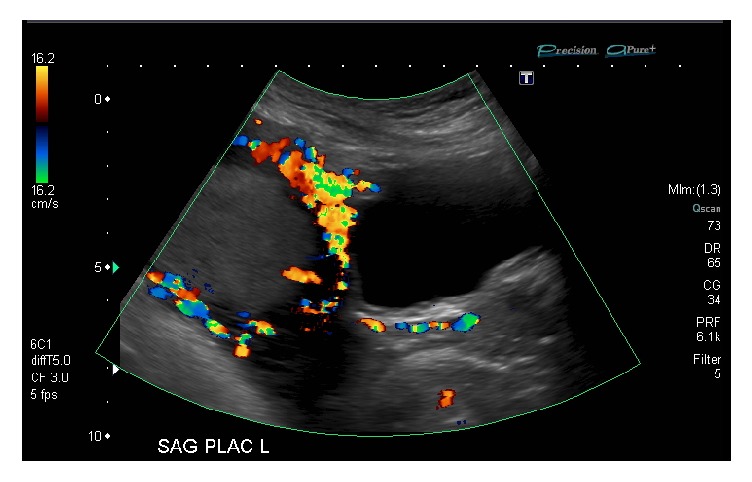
Doppler ultrasound in the sagittal plane at midline in the pelvis demonstrates turbulent peripheral vascularity in the placenta extending across the myometrium to the posterior wall of the bladder. The bladder contour is otherwise smooth; however, the finding remains highly suggestive of placenta percreta. No defect in the uterine wall could be identified, but given the large volume of hemorrhagic ascites, an emergency diagnostic laparoscopy was subsequently performed.
